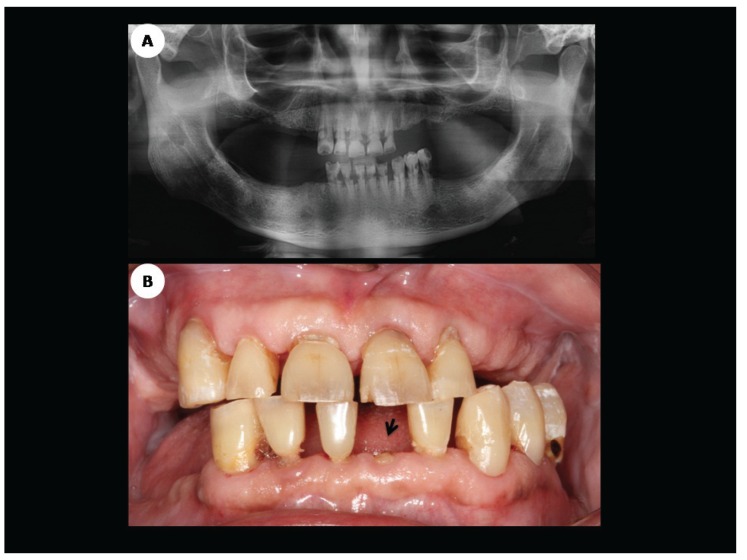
A. Mandible panoramic radiography showing multiple teeth affected by advanced cervical radiation-related caries; B. Clinical aspect of the same patient shown in 1A, demonstrating an apparent incipient case of RRC. Note dental crown amputation of the left mandible central incisor following a few days of the radiographic assessment (arrow).
